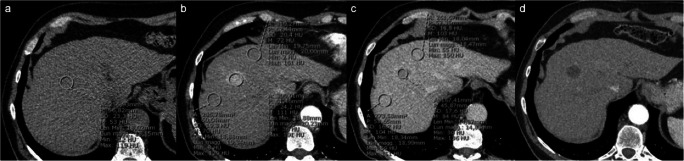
Tracing method of ROIs for the quantitative analysis. In order to compute CER, LLC, WO_abs_, WO_rel_, and DPAR, by each reader, one ROI was manually placed on the lesion in the unenhanced (a), arterial (b), and delayed (c) phase and two ROIs were placed on the adjacent hepatic parenchyma, excluding visible hepatic and portal vessels, bile ducts, and artifacts, both in the arterial (b) and delayed (c) phase. For the well-defined nodule showed in the example, the following quantitative parameters were calculated: CER 109.4, LLC 56.3, WO_abs_ 46.5, WO_rel_ 24.3, and DPAR 123.2. The 1-month follow-up CT scan shows CR, without any intratumoral arterial enhancement (d)
